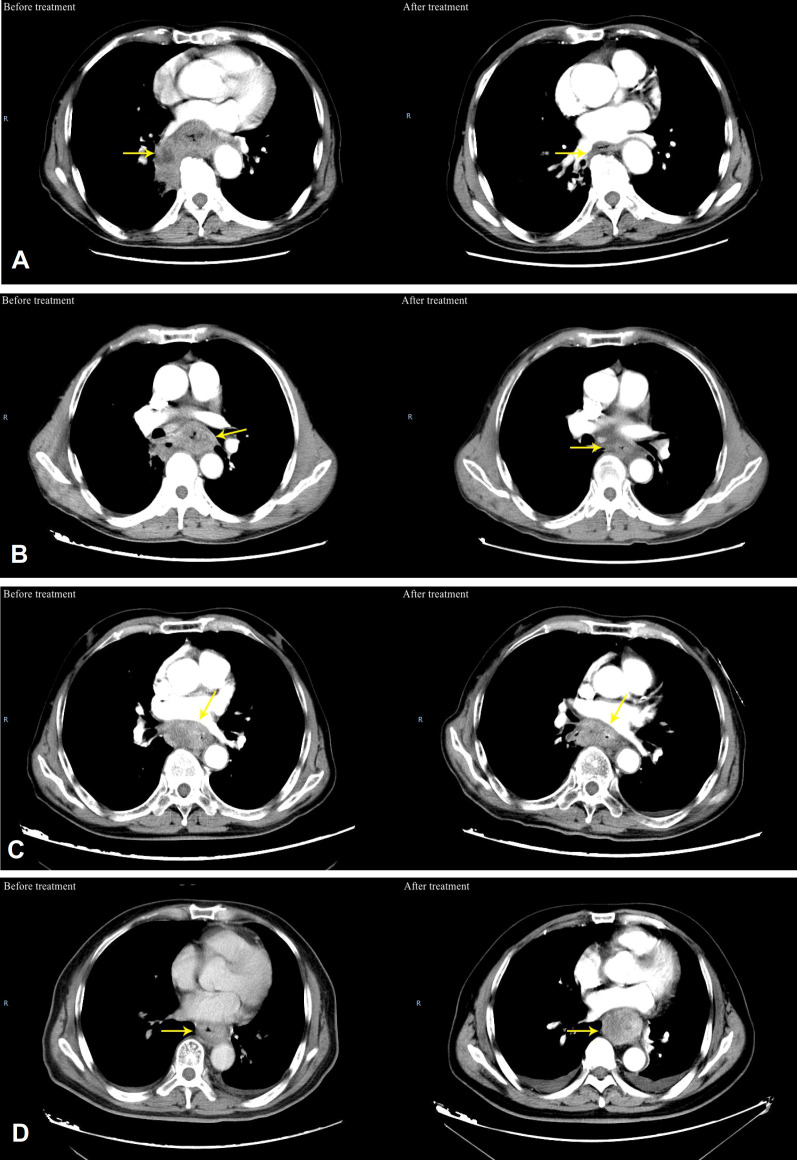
Typical patients of efficacy evaluation before and after treatment for ESCC. (A) CR, which means the typical patients of efficacy evaluation before and after treatment for ESCC, (B) PR, which means that typical patients of efficacy evaluation before and after treatment for ESCC; (C) SD, which means that the sum of esophageal tumor diameters decreased by < 30% on CT after treatment; (D) PD which means that the sum of esophageal tumor diameters increased by ≥20% on CT after treatment).
